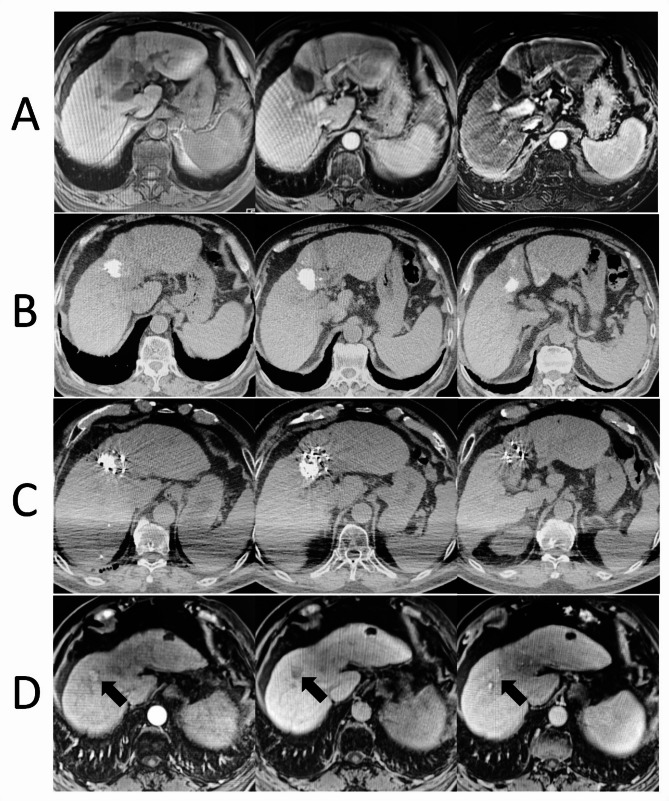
A 71-year-old male patient. In January 2023, a CT scan performed for pneumonia revealed a hepatic segment IV mass (4.2 × 3.3 cm). Biopsy confirmed hepatocellular carcinoma. In February 2023, the patient underwent transcatheter arterial chemoembolization (TACE) and radiofrequency ablation (RFA), followed by oral lenvatinib for anti-tumor therapy. MRI and CT scans in October 2023 showed post-intervention changes (A and B). However, a significant increase in serum AFP levels suggested local tumor recurrence. In November 2023, based on the patient’s strong preference, a CT-guided ^125^I particle implantation was performed (C). Three months post-implantation, the tumor size remained unchanged, but serum AFP levels significantly decreased. In November 2024, MRI revealed a new nodule in hepatic segment VIII, indicative of intrahepatic metastasis (D, black arrow). The patient achieved partial response three months after ^125^I particle implantation, with a local progression-free survival (LPFS) of 12 months and an overall survival (OS) of 13 months
